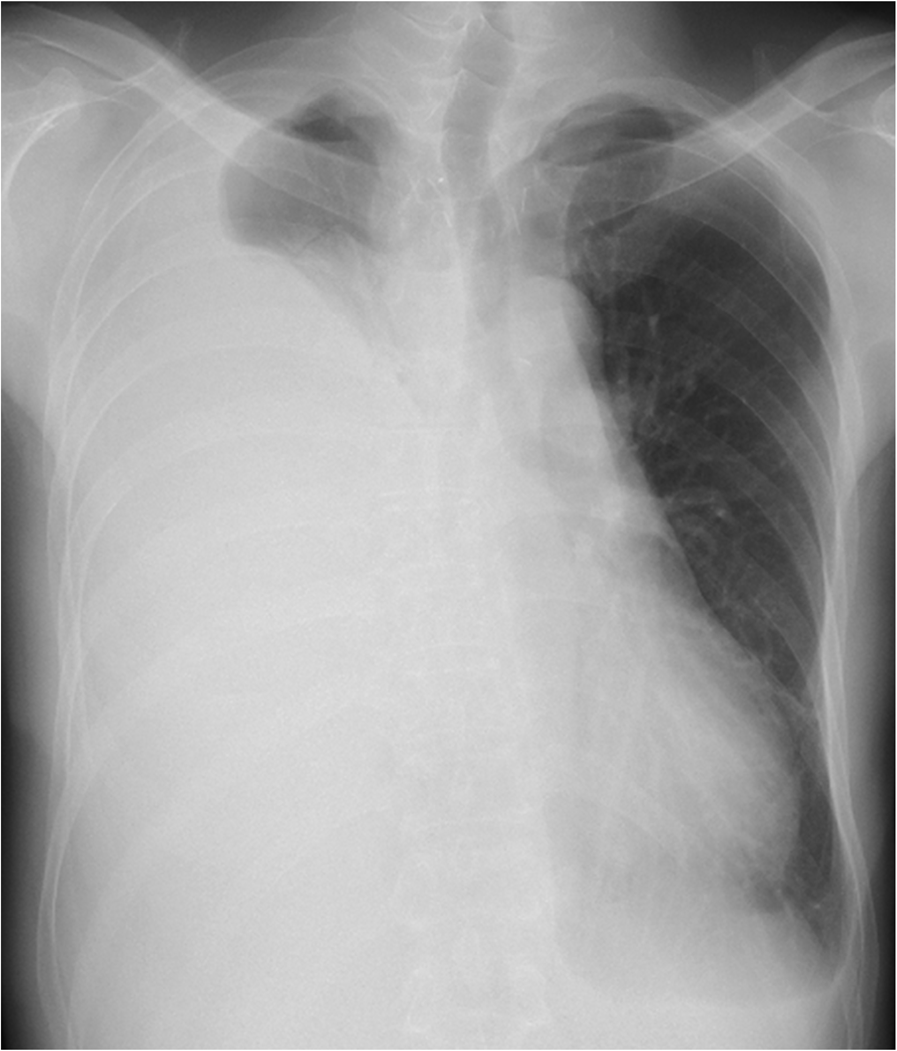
Chest X-ray 1 month after operation revealed large amounts of right-sided pleural effusion
